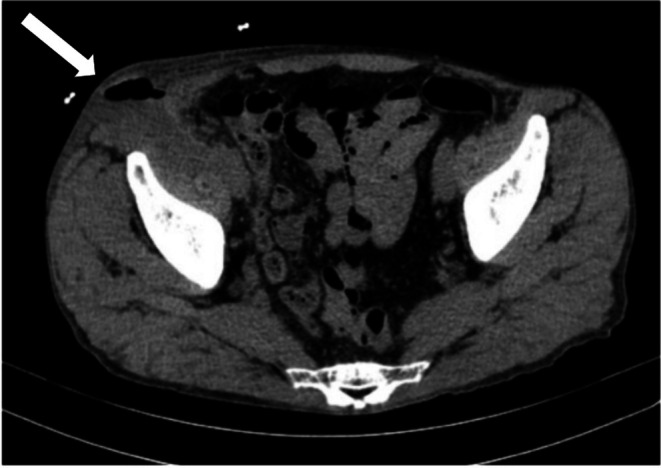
Plain abdominal CT scan. A low‐density area (white arrow) extending from the retroperitoneum near the cecum to the right inguinal region can be observed, suggesting abscess formation.
